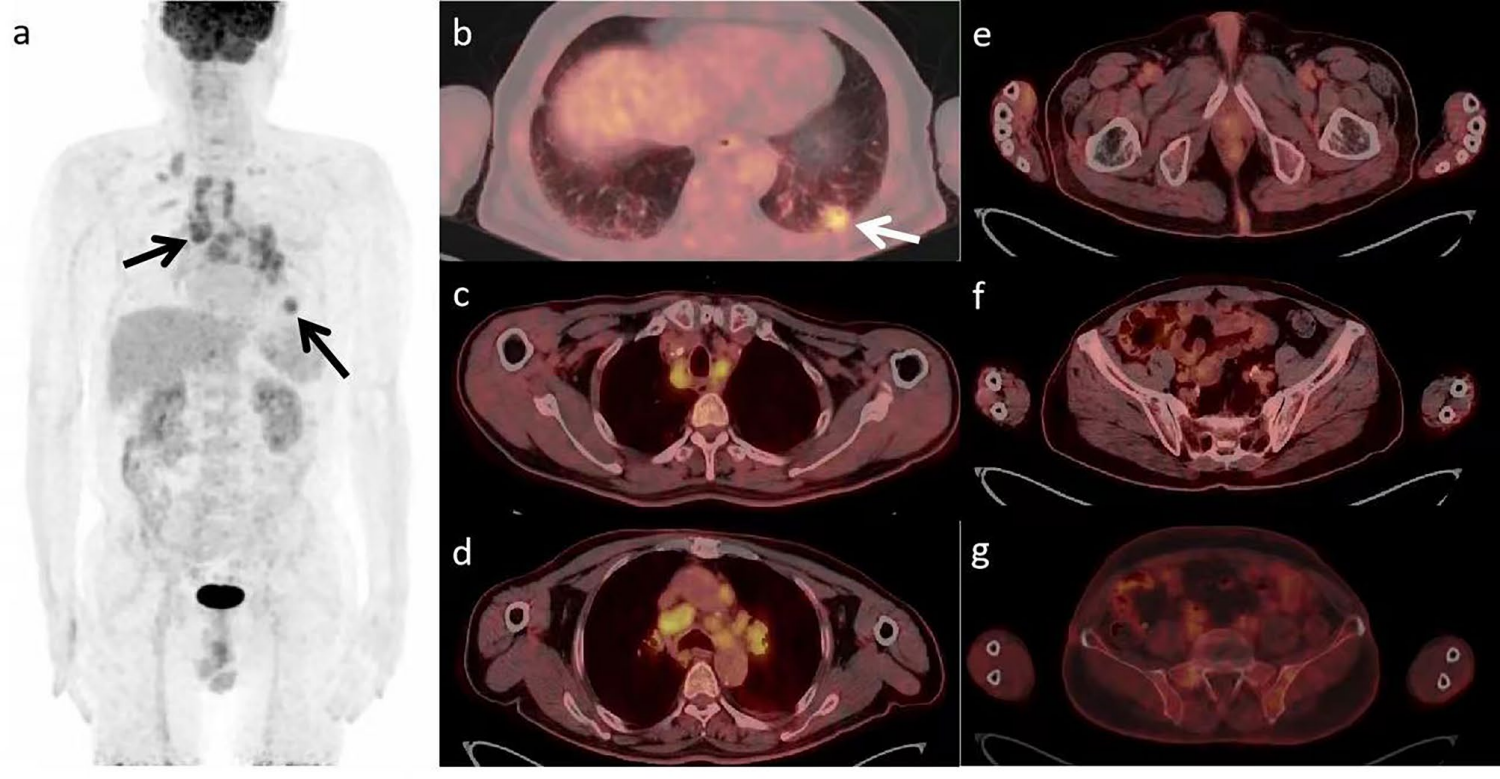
^18^ F-FDG PET/CT images. a Maximum intensity projection (MIP) image showed a increased FDG uptake in left pulmonary nodule and multiple mediastinal metastatic lymph nodes (arrows). b Axial PET/CT fused image showed a hypermetabolic left pulmonary nodule, about 2.4 × 1.9 cm, Maximum Standardized Uptake Value (SUVmax) 5.7 (arrow). c,d Hypermetabolic multiple mediastinal metastatic lymph nodes, SUVmax 4.5-7.0. e,f,g There were no obvious hypermetabolic foci in prostate, pelvic lymph node and pelvic bone
